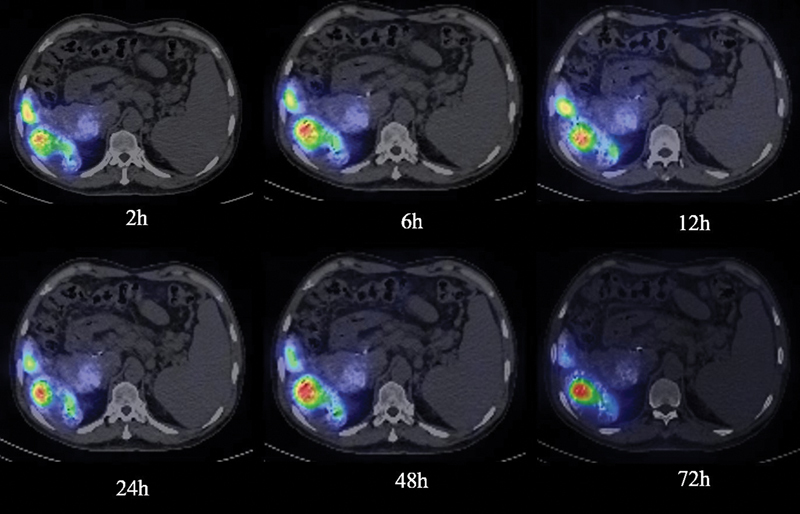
Co-registered single-photon emission computed tomography/computed tomography images of liver up to 72 hours of above-mentioned patient in Fig. 6 after transarterial administration of Rhenium-188-N diethyldithiocarbamate, showing high retention of activity in seg VI lesion.
